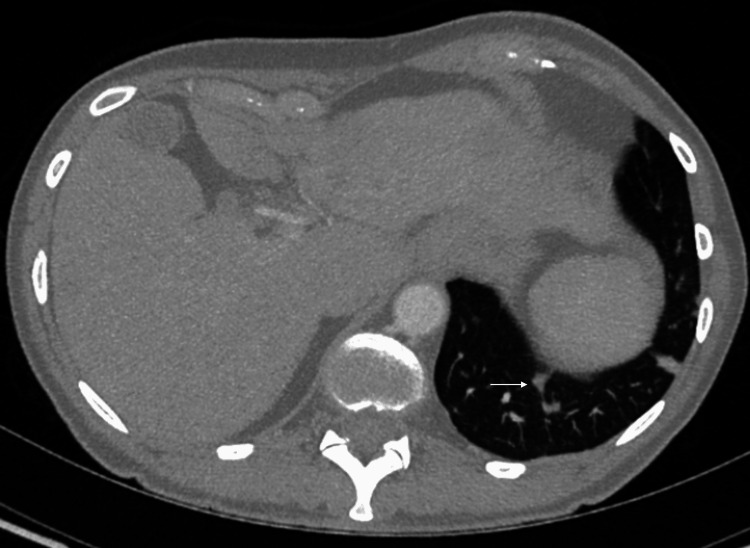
Repeat axial CT showing left lung base lesion demonstrating decreased diameter in May 2020
